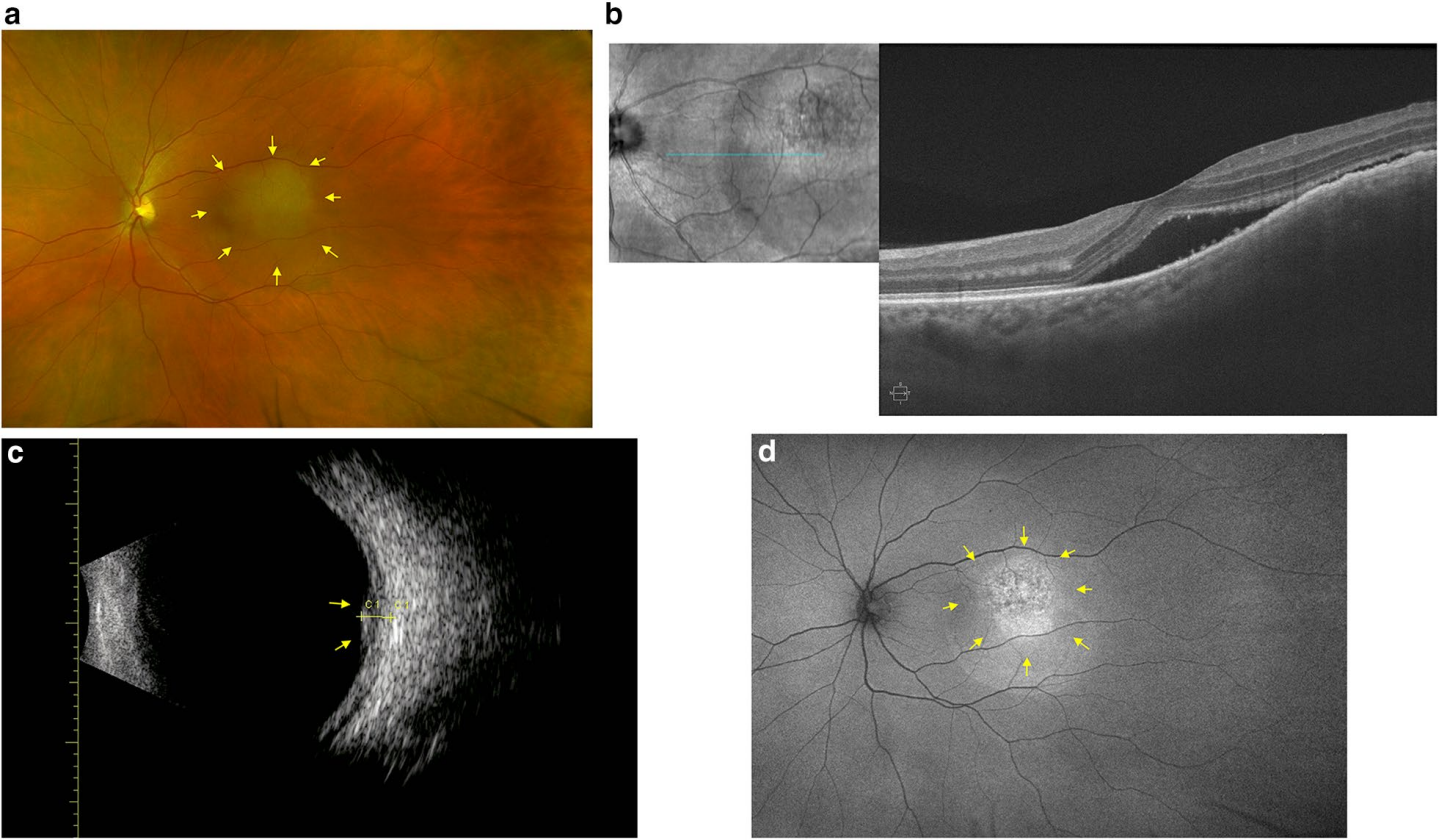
Imaging studies performed in June 2017 for a 58-year-old female with choroidal metastasis from primary breast carcinoma. a The extent of the yellow-colored choroidal mass superior and temporal to the macula is marked (yellow arrows). b Structural OCT demonstrated subretinal fluid associated with the choroidal mass. c Ultrasound showed a 2.47 mm choroidal lesion (yellow arrows). d Fundus autofluorescence demonstrated a hyper-fluorescent lesion (yellow arrows) with surrounding subretinal fluid
